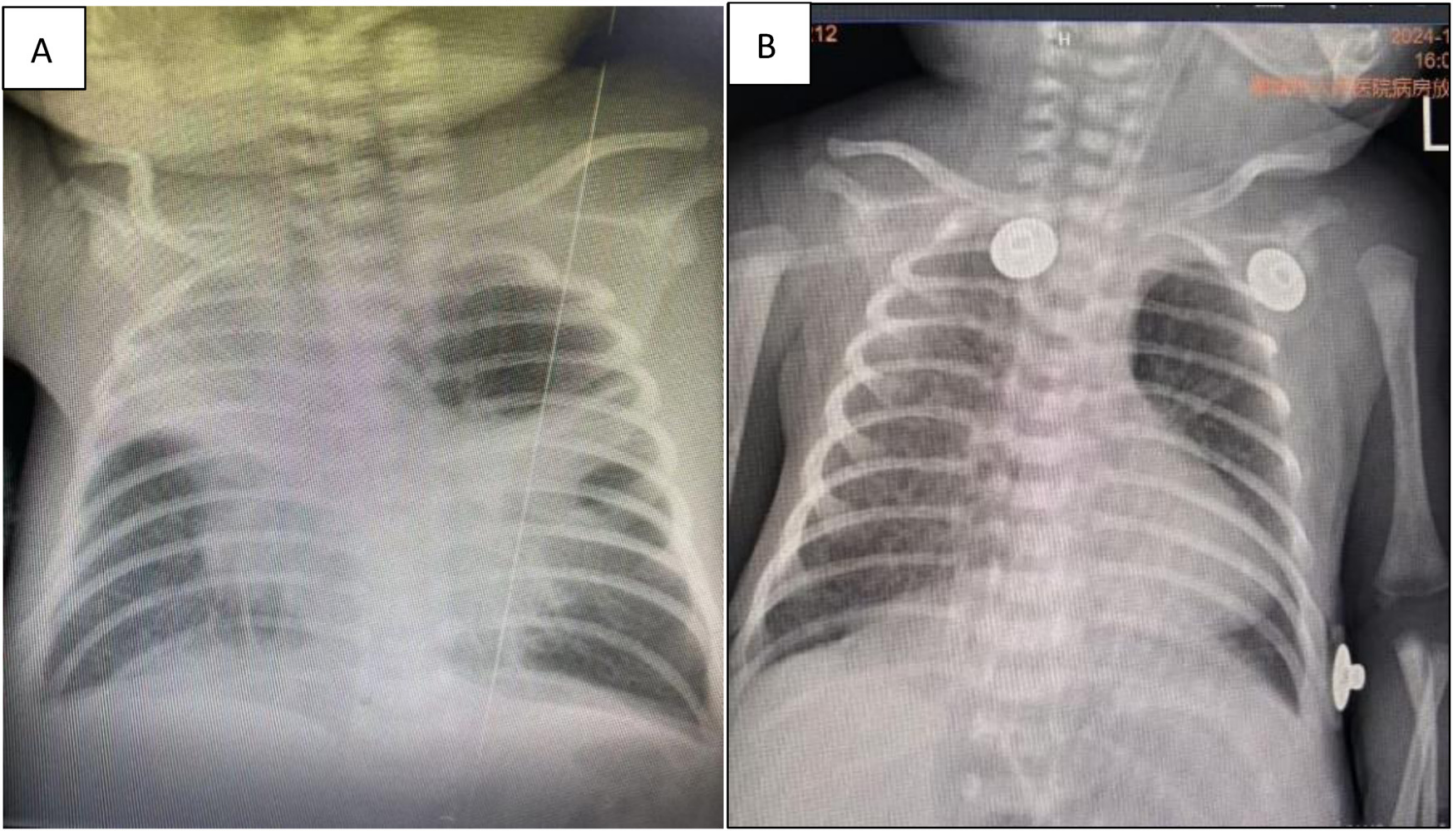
(A) Atelectasis of the left lung in chest radiographs. (B) Atelectasis after bronchoscopic lavage treatment during follow-up in chest radiographs.
